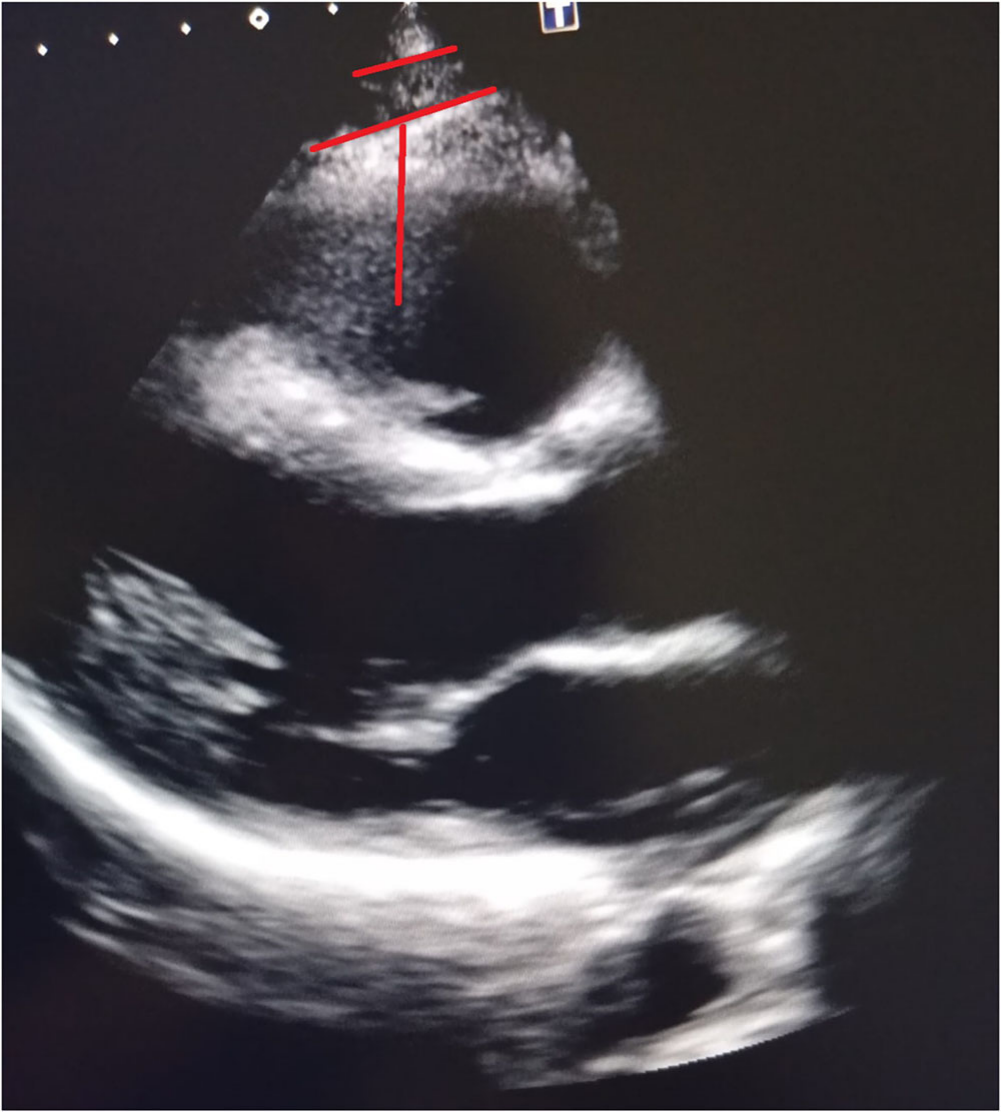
Measurements of epicardial fat thickness through transthoracic echocardiography. In the long‐axis parasternal view of a transthoracic echocardiogram, the red lines indicates epicardial fat located over the free wall of the right ventricle. It appears as an echo‐free space between the outer myocardial wall and the visceral layer of the pericardium.
